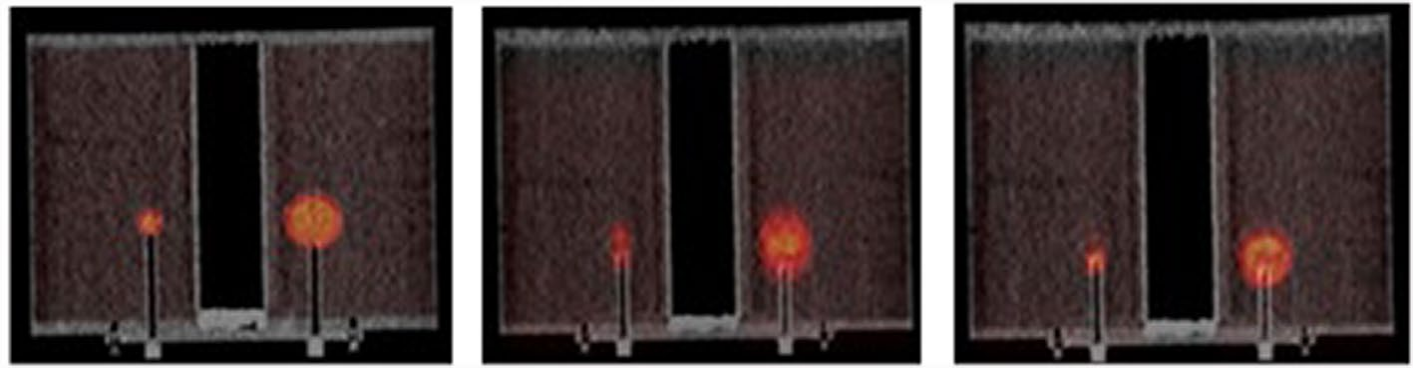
Fused 3D PET/CT image, for a static image (left), motion amplitude 30 mm (middle and right) without and with DDMC, TBR 5
